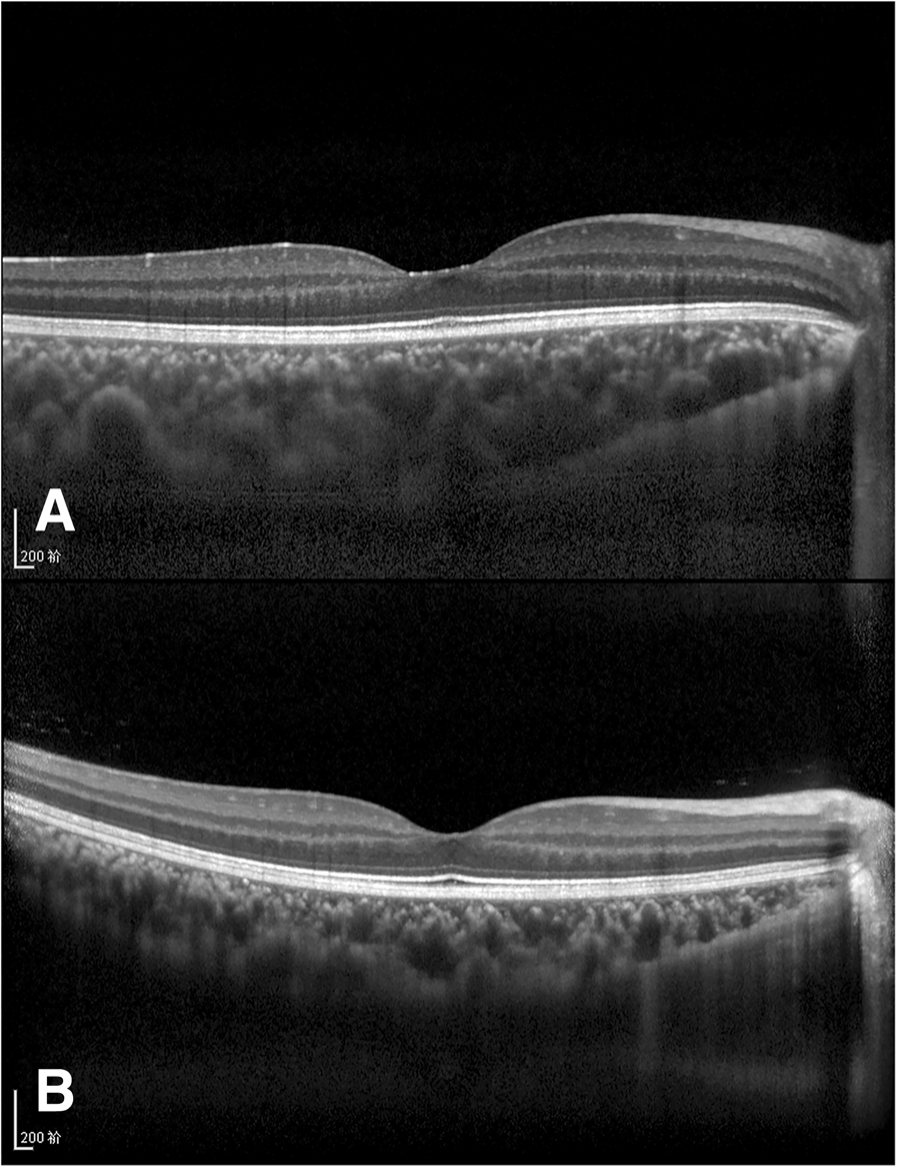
EDI-OCT images from a patient with NS (a) and a normal child (b). The CT of the NS patient was much greater than that of the normal child
